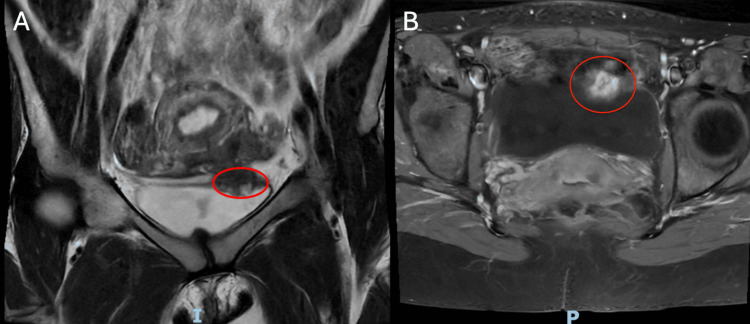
Pelvic MRI demonstrating 2.4 x 2.1 x 2.7 cm bladder dome lesion. (A) Coronal T2-weighted MRI demonstrating a hypointense lesion at the left bladder dome (red circle). (B) Axial T1-weighted MRI demonstrating multiple foci of hyperintensity (hemorrhagic content) within the lesion (red circle).
